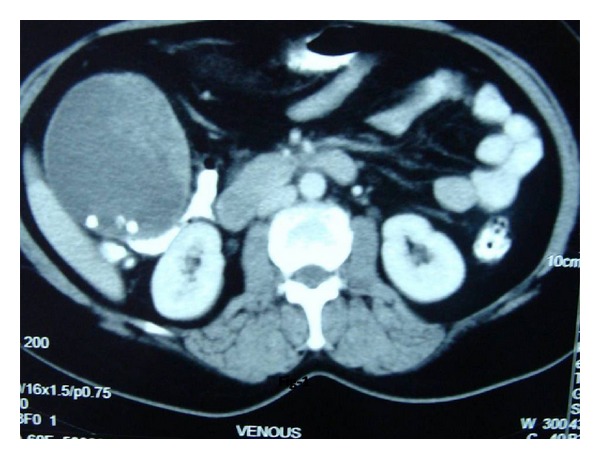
Biphasic CT abdomen showing gallbladder grossly distended: soft tissue mass in gallbladder with multiple intraluminal projections and features suggestive of cholelithasis with polypoidal gallbladder malignancy.
